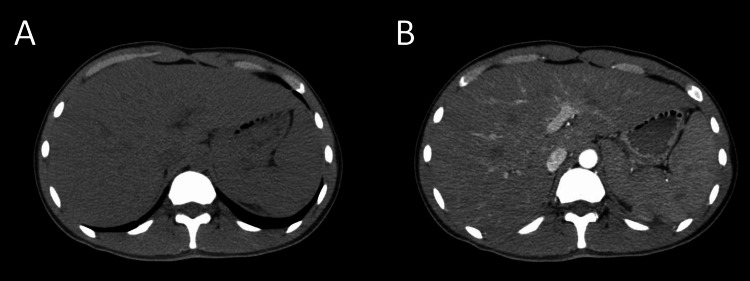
CT scan of the abdomen with IV contrast. (A, B) Axial sequences of the abdomen showing hepatomegaly and splenomegaly before and after IV contrast. CT: computed tomography. IV: intravenous.
